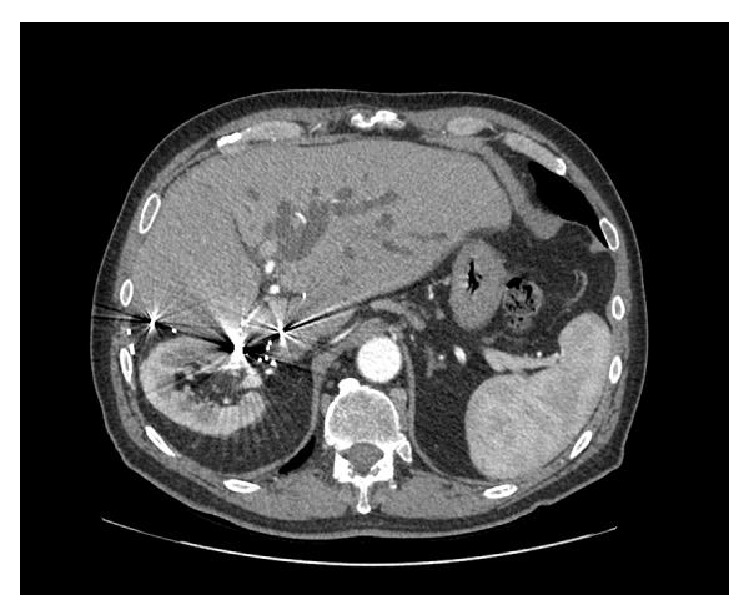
Axial contrast-enhanced CT displaying dilatation of the left hepatic duct due to an enlarging central metastatic lesion.
